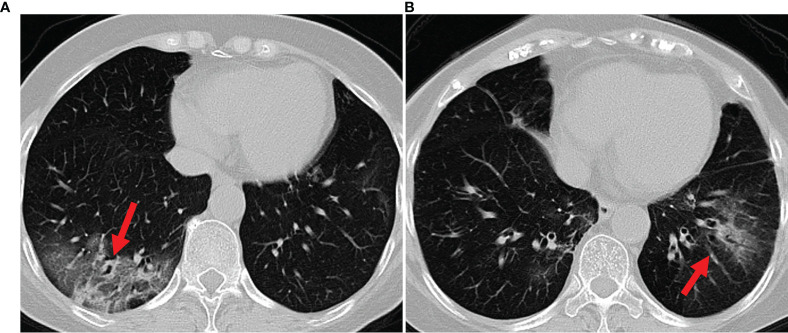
Example axial CT slices of viral pneumonia (A), bacterial pneumonia (B). Accurate classification of different types of pneumonia is imperative for timely diagnosis and treatment. However, viral pneumonia and bacterial pneumonia display similar appearances in a CT image, which makes it difficult to accurately differentiate a patient with viral pneumonia from a case of bacterial pneumonia.
